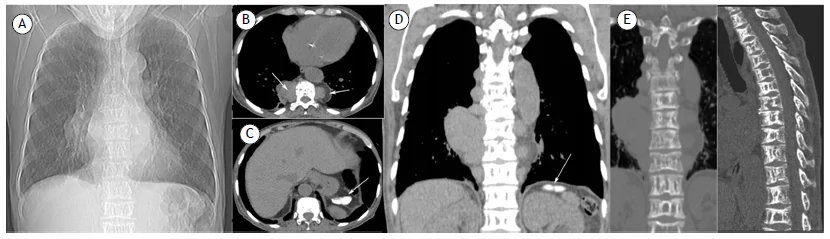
In A, posteroanterior chest radiograph showing bilateral paravertebral masses. In B-D, chest CT images confirming the presence of multilobulated, heterogeneous masses with soft-tissue density and areas of fat inside (-45 HU; arrows in B). Note also the significant reduction in the volume of the spleen, which appears calcified (arrows in C and D). In E and F, anteroposterior and lateral radiographs of the thoracic spine showing changes in the vertebral body structure consistent with sickle cell anemia.
